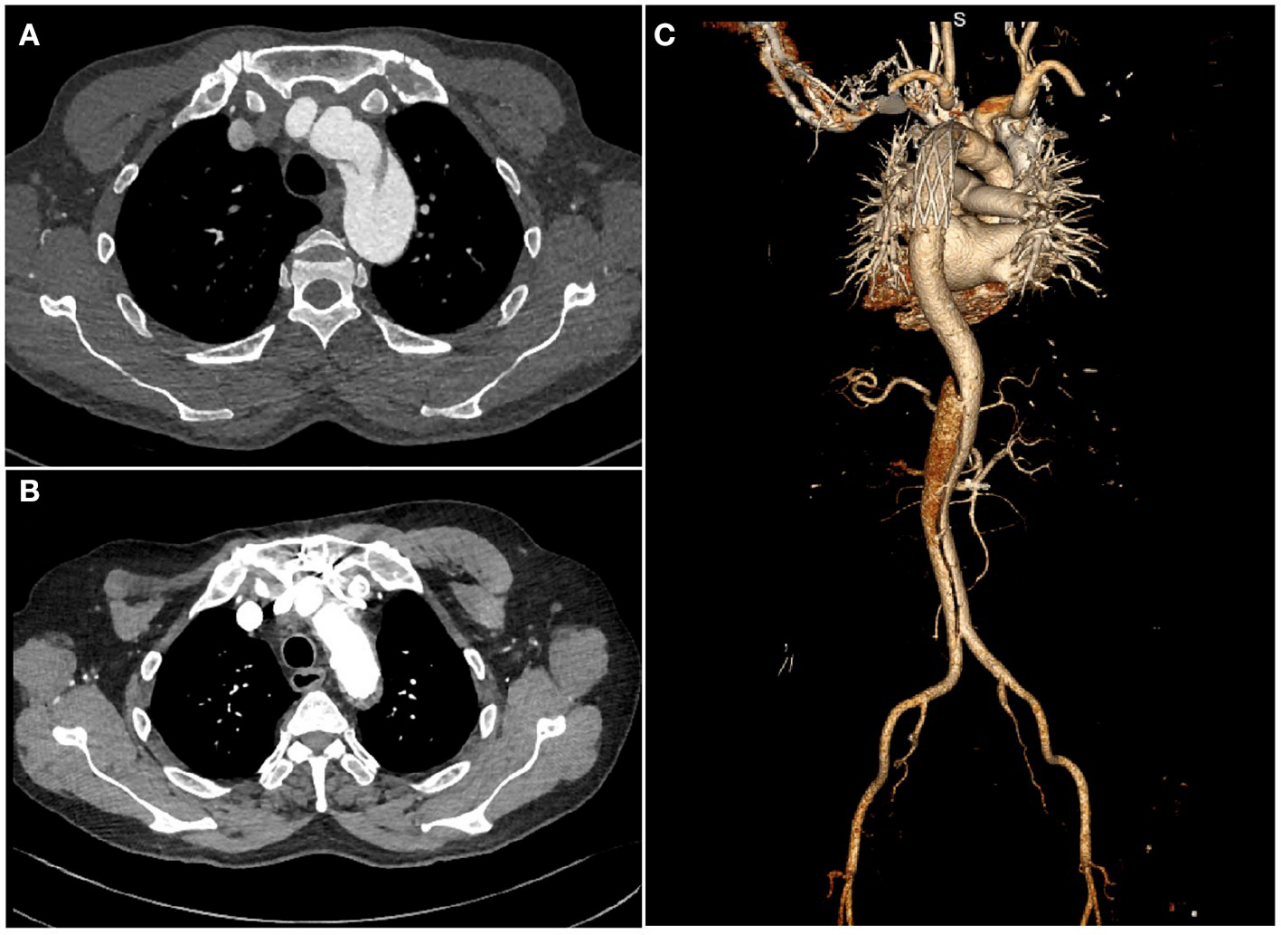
Computed tomography images of a patient with chronic type B dissection with aortic arch involvement (A) preoperatively (B,C), postoperatively.
